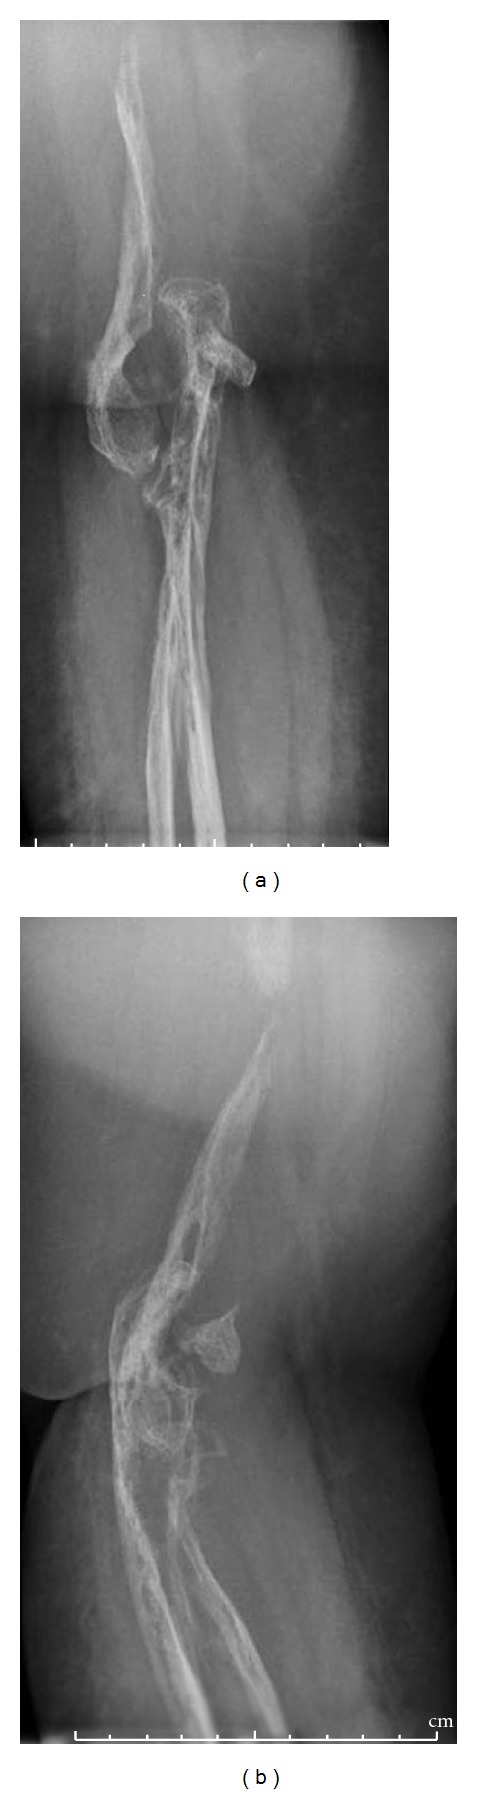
Anteroposterior and lateral plain radiographs of the elbow showing dislocation of the humeroulnar joint and fragmentation and disappearance of the distal humerus.
